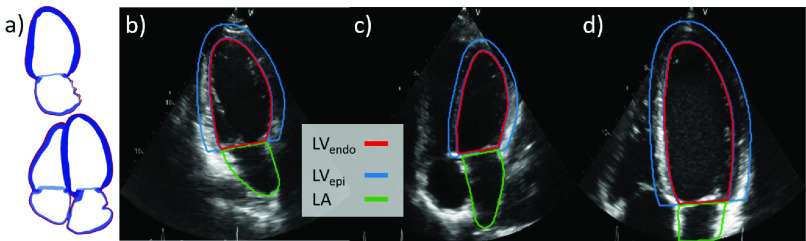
Example application: echo segmentation. a) Apical two chamber (top) and apical four chamber (bottom) views as shown in an anatomical model. The right images show example real apical two chamber (b) and apical four chamber (c, d) echo images with task labels. (b, c) show the full heart while d) is zoomed to focus on the left ventricle.
